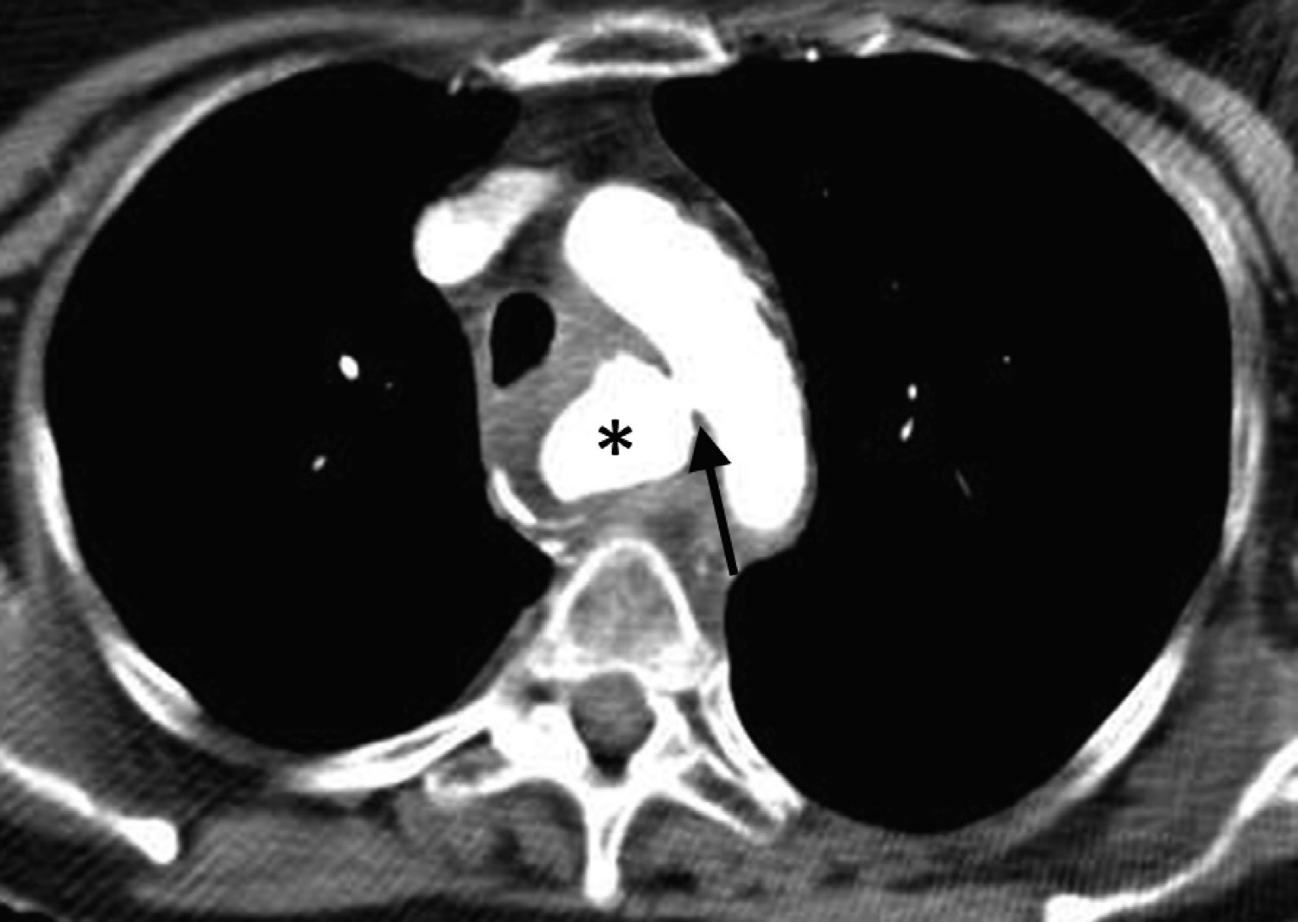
CT angiography showing contrast extravasation into the esophagus (star) from a fistula extending from the medial aspect of the aortic arch (arrow).
